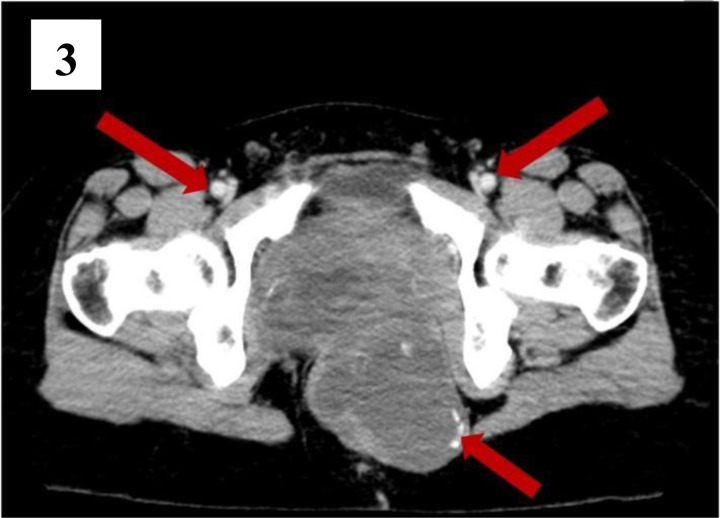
is an axial view of the contrast CT scan showing the contrast enhanced vessels (red arrows above the image) and foci of coarse calcification in the periphery of the cystic component (red arrow below the image).
